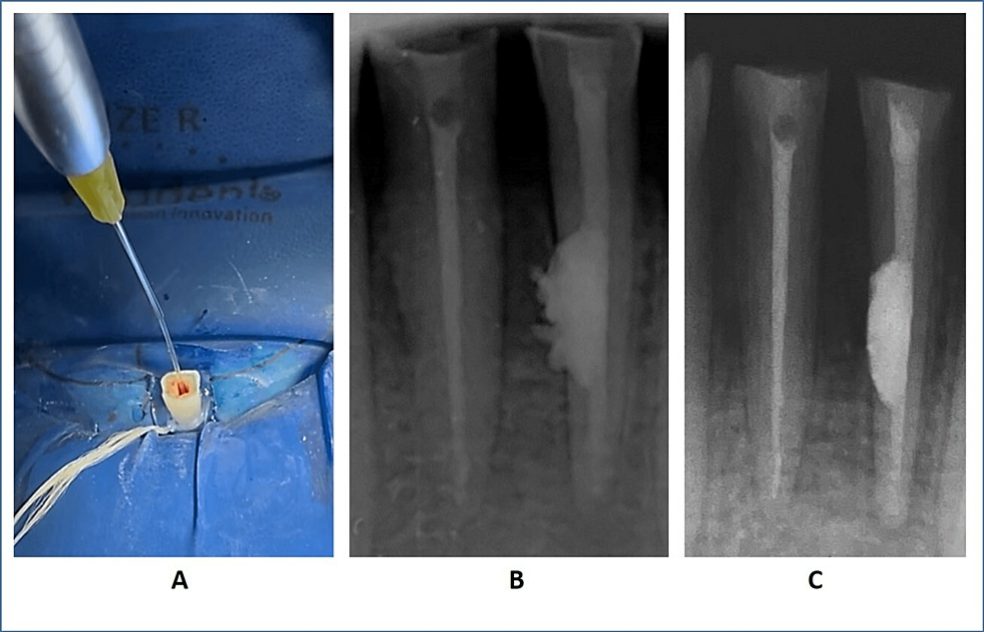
Postoperative assessment with a follow-up radiograph. A: Laser debridement. B: Immediate postoperative radiograph; C: Six-month follow-up radiograph.
